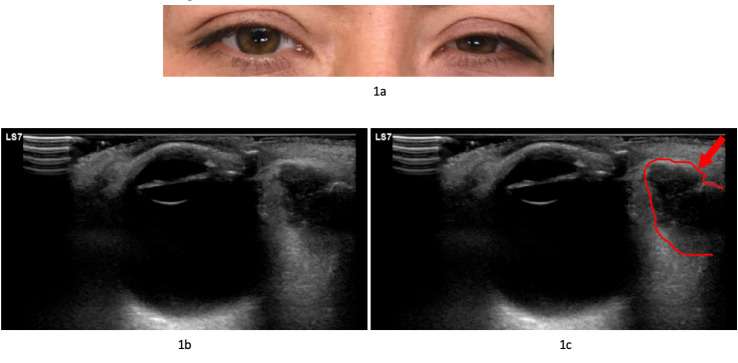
a) Appearance of the patient’s eyes on presentation to the Emergency Department; b) and c) Sonographic appearance of orbit with left lateral rectus muscle. Mass denoted in 1C by red arrow and red outline.
